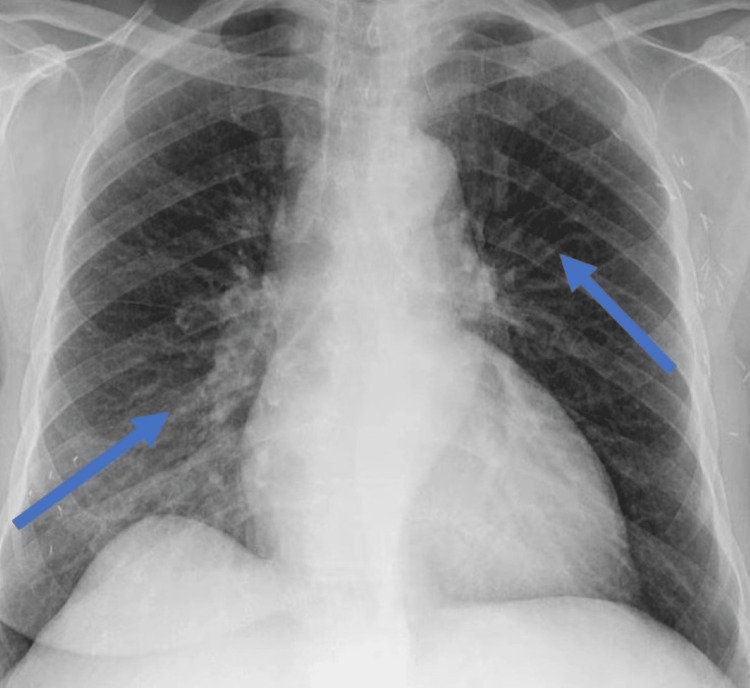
Chest x-ray showing prominent vascular marking suggestive of acute pulmonary edema (blue arrows).
